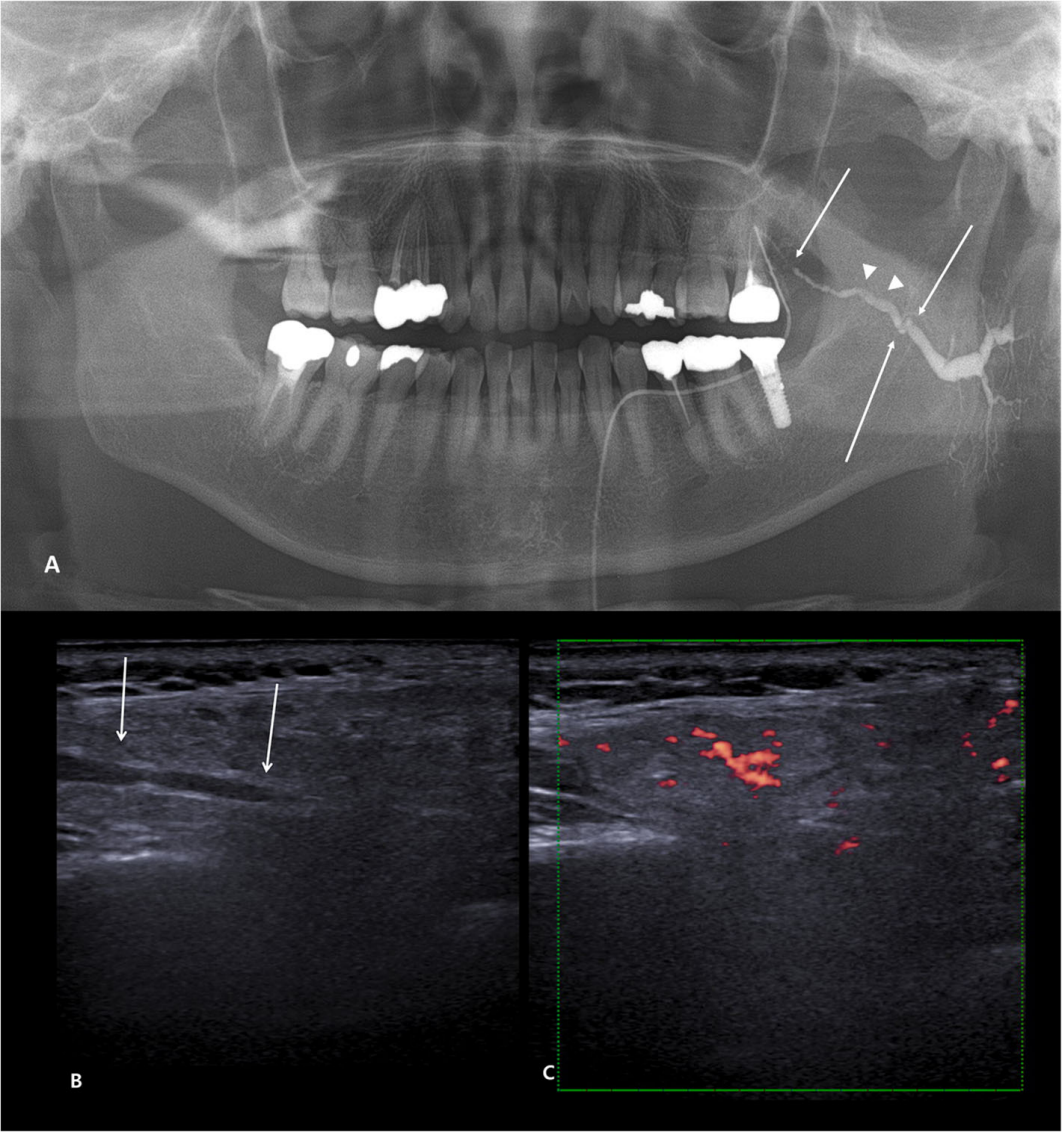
Representative sialography and ultrasonography of patients diagnosed with sialadenitis. a Left Stensen’s duct and intraglandular ductile show dilation with stenosis (arrows). Note the irregular surface of ductal filling (arrowheads) suggesting of plugs and coagulated substances. b Dilated duct is shown on ultrasonography of the same patient (arrow). c Power Doppler image shows increased vascularity, implicating inflammatory state of parenchyma
